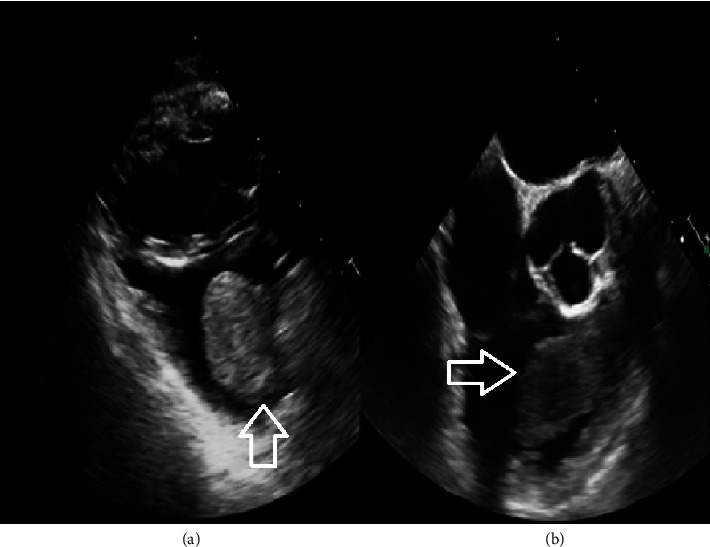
Transesophageal echocardiographic views of the RV mass. (a) Shows trans-gastric views which demonstrates the attachment of the mass to the undersurface of tricuspid annulus adjacent to the anterior tricuspid leaflet (white arrow). (b) Shows the short axis view where the RV mass (white arrow) is visualized traversing the pulmonary valve.
